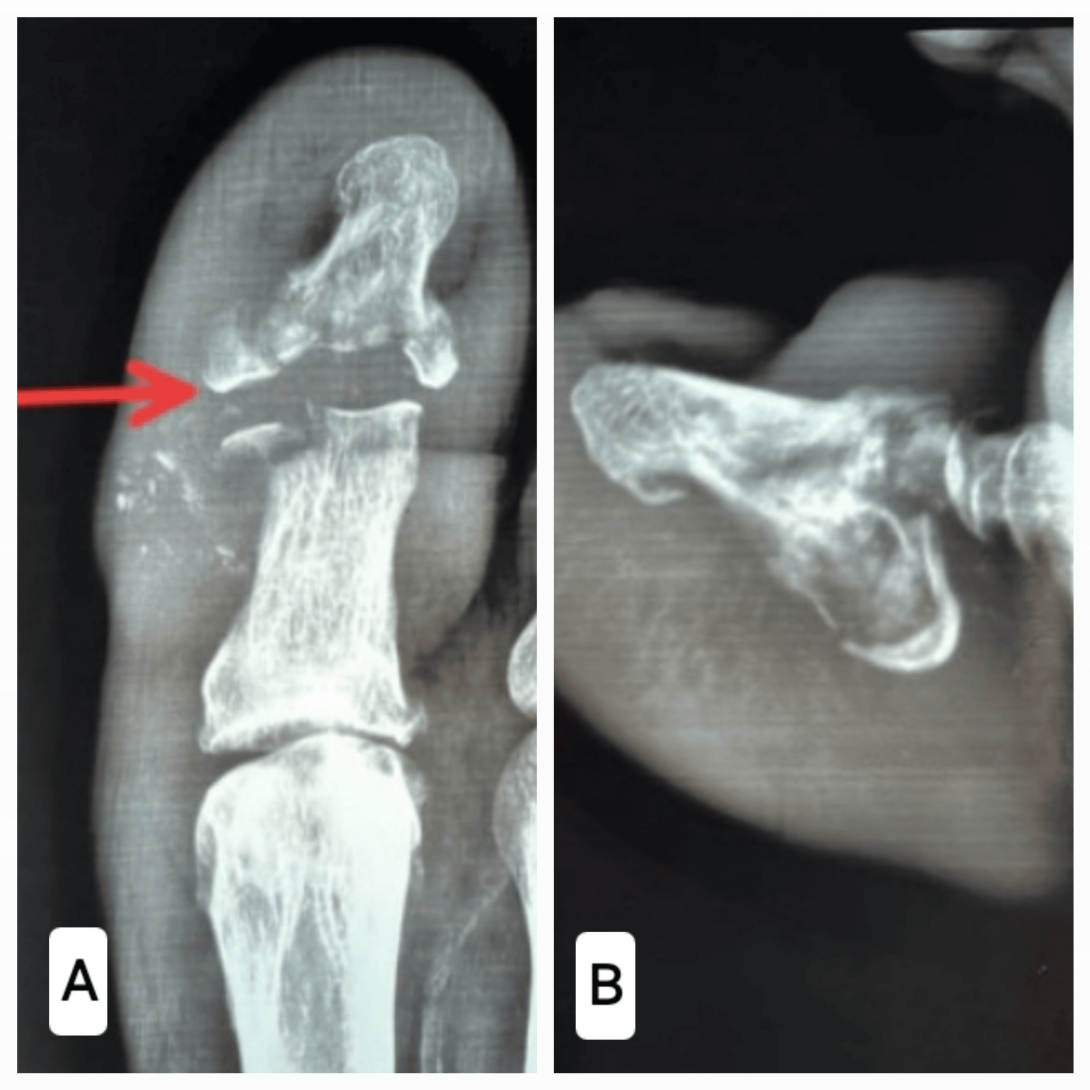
Plain radiograph of the affected great toe. A: The antero-posterior view showing intra-articular fracture of distal phalanx with fragmentation and bony debris. B: The lateral view showing intra-articular fracture of distal phalanx.
